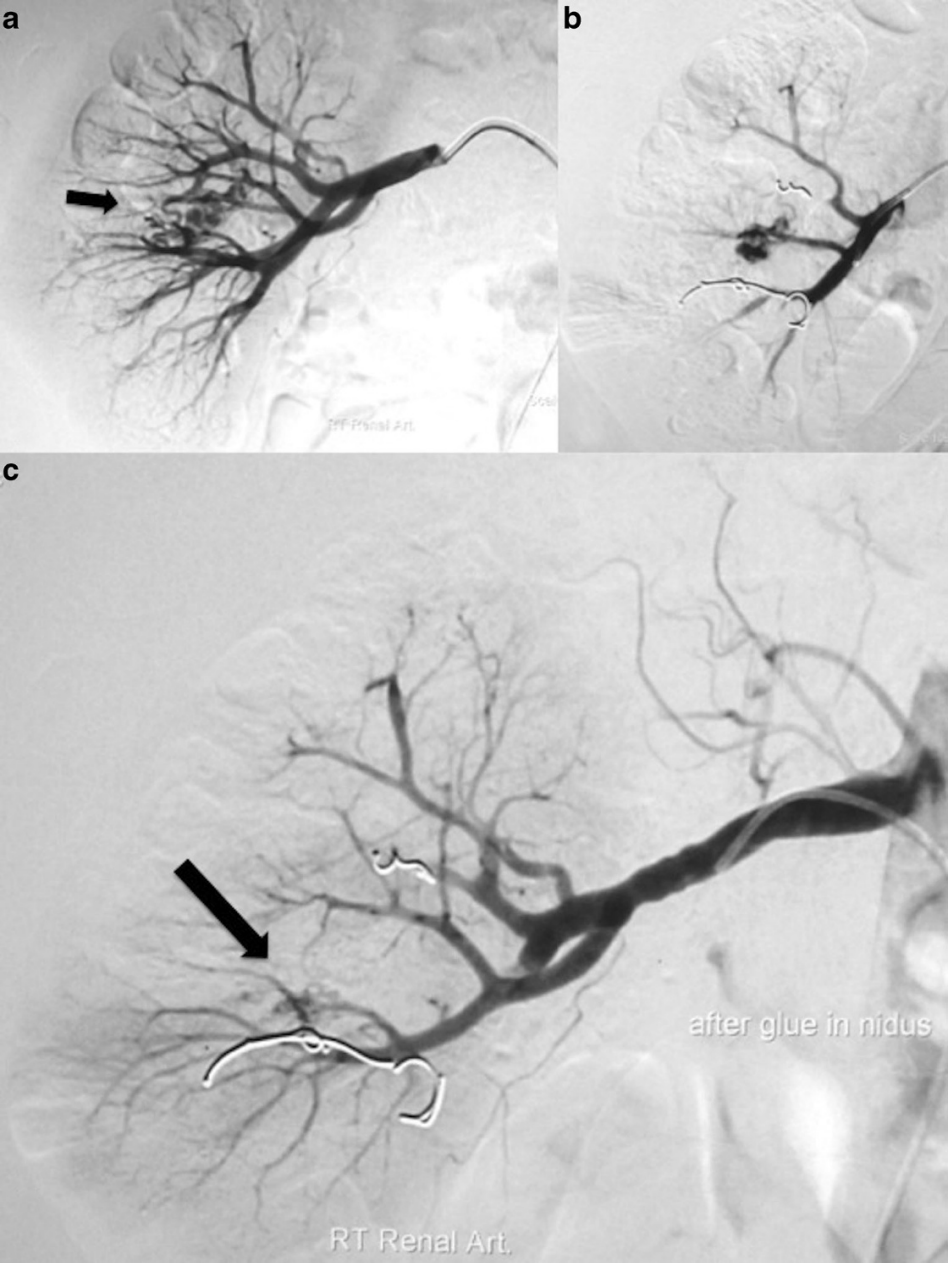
A 33-year-old man was admitted to the emergency department because of acute right flank pain and severe macroscopic haematuria and laboratory findings like the case described in Figs. 1 and 2. Contrast CT scan revealed a “bag-of-worms”-like mass in the middle portion of the right kidney (not shown). a Emergency angiography with selective catheterisation of the right renal artery confirmed an area of tortuous, coiled vascular channels with early filling of the renal vein within a few seconds after contrast injection in the centre of the kidney (arrow). These findings confirmed the RAVM diagnosis, which appeared to receive blood supply from three small arterial branches. b Two of the feeding branches were successfully embolised with metallic coils of 3 × 2.6 (*n* = 4) mm diameter (2D Helical-35, Boston Scientific, Cork, Ireland). c The middle feeding branch was closer to the nidus (arrow), and embolisation by injection of tissue NBCA glue (GlueBran2, GEM S.r.l., Viareggio, Italy) was chosen. The final angiogram showed a good post-embolisation result. The haematuria stopped and the patient recovered within the next days
